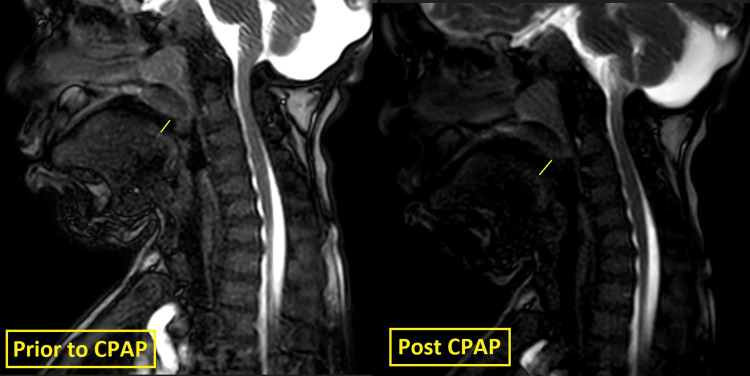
Cine MRI of a patient for titration of continuous positive airway pressure (CPAP) Prior to CPAP, the dimension was 4. Post CPAP, the dimension increased to 6.1. Images are obtained prior and post application of CPAP to show the improvement of minimum anteroposterior dimension (represented by yellow lines) of the oropharynx, which is more than 50%.
